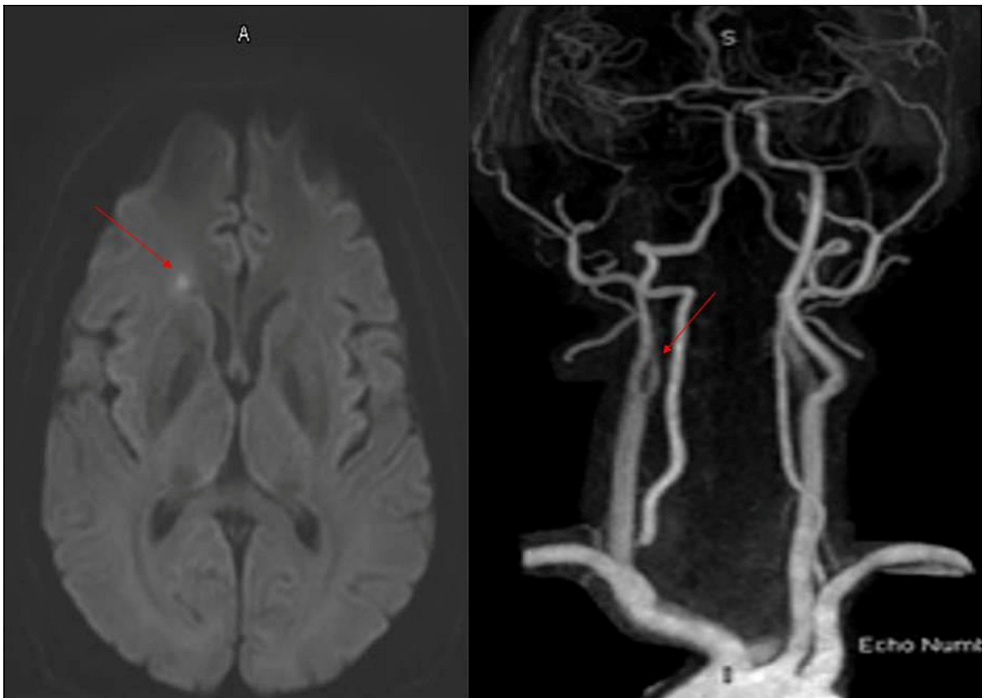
MRI head diffusion-weighted image (DWI) and MRA head and neck vessels with gadolinium MRI head DWI sequence showed a small right deep frontal white matter region acute ischemic stroke (as shown by the red arrow in the left panel). MRA (magnetic resonance angiography) head and neck vessels with gadolinium showed tapering of the right internal carotid artery (ICA) just after carotid bifurcation and proximal segment of left internal carotid artery followed by distal occlusion along the rest of its neck and intracranial segment (as shown by the red arrow in the right panel).
